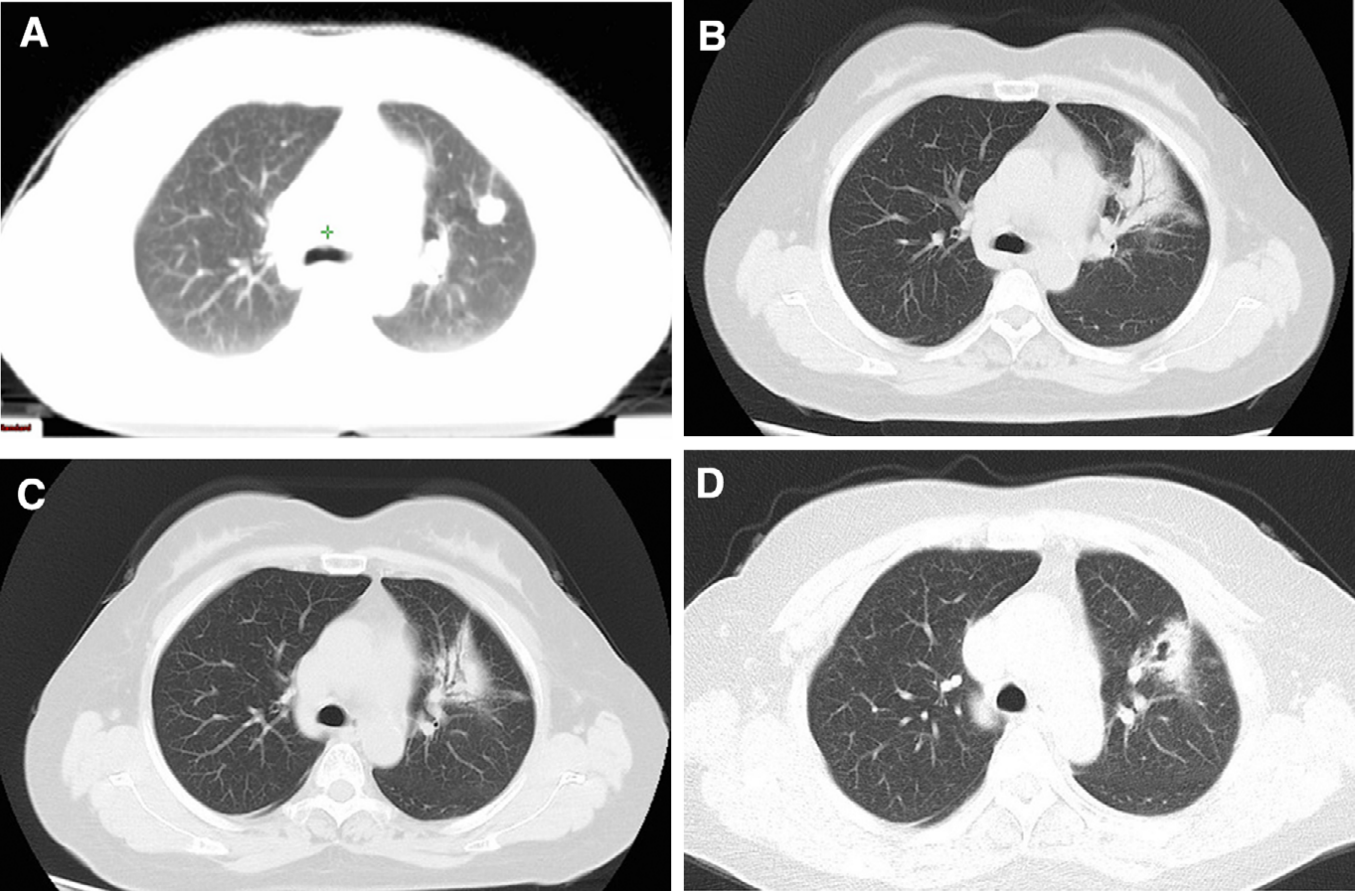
The chest CT images of the patient with grade 2 radiation induced pneumonitis (A: pretreatment image; B: 2 months after radiotherapy; C: 8 months after radiotherapy; D: 22 months after treatment) [Color figure can be viewed at http://wileyonlinelibrary.com]
